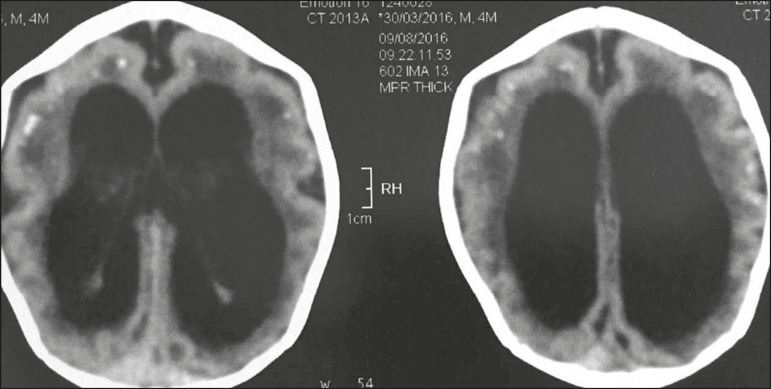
Non-contrast-enhanced computed tomography of the brain in a four-month-old
